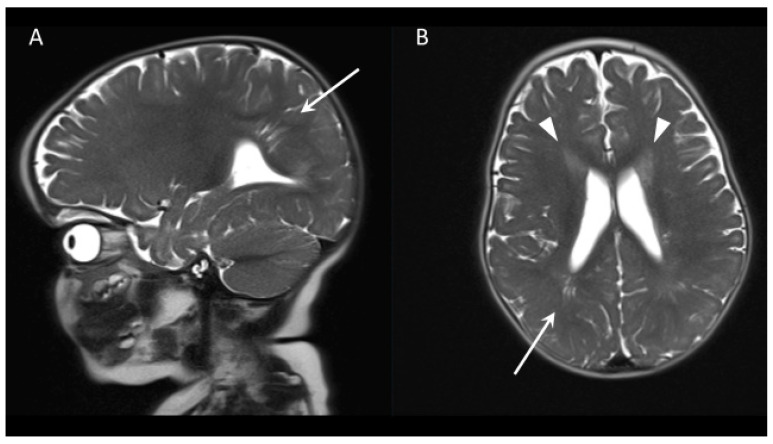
Cranial MRI at 10 months of age. T2-weighted fast spin-echo sequence in (A) sagittal and (B) axial orientation. Right occipital stria-like enlarged Virchow–Robin spaces (arrow). T2-hyperdensities rostral to the ventricles (arrowheads).
